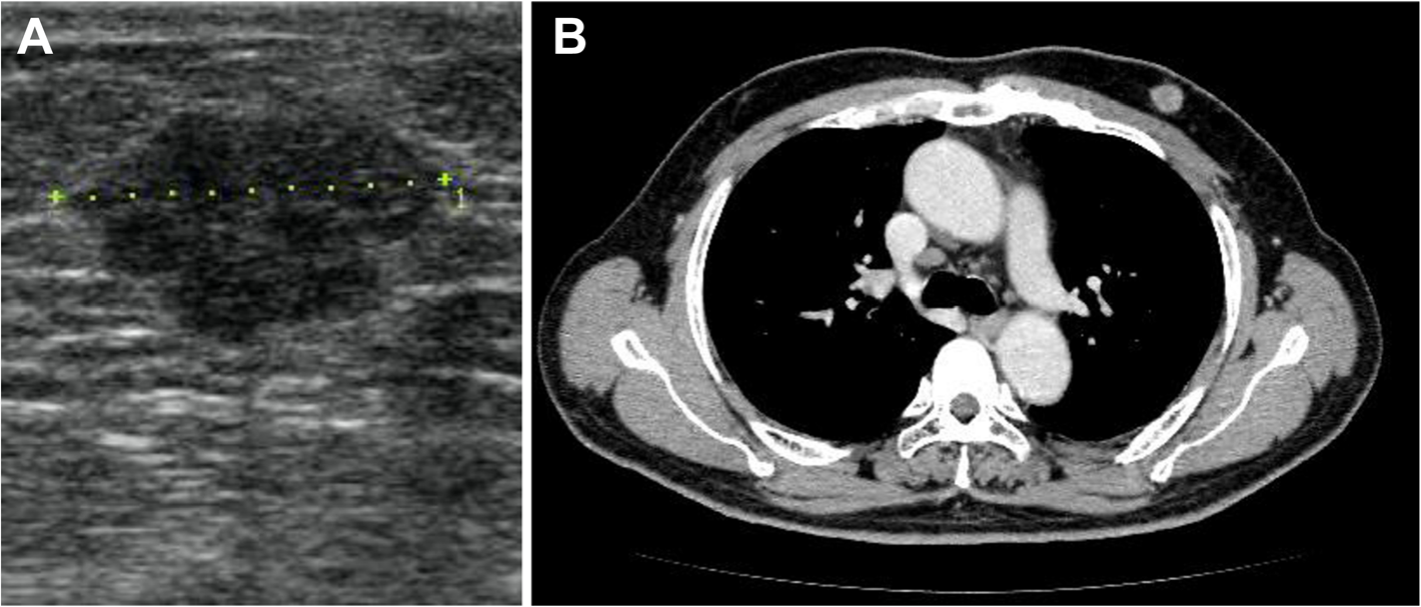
a A preoperative US scan showing a round mass of 16 mm in diameter in the left subareolar area. b A preoperative chest CT scan showing a round tumor of 16 mm in diameter in the left subareolar area
